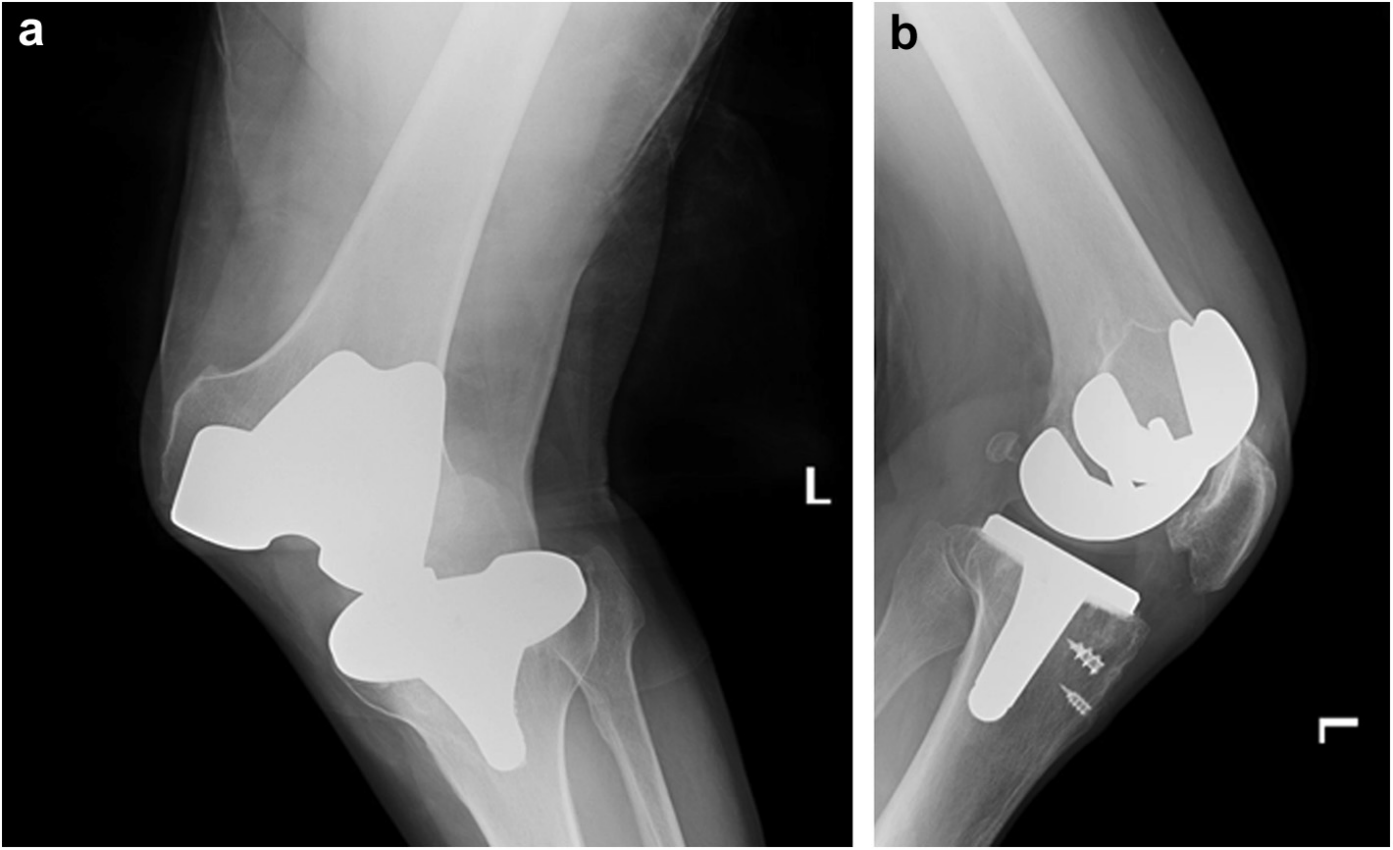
Anteroposterior (a) and lateral (b) radiographs demonstrating a left lateral TKA dislocation. Also noted are Arthrex Corkscrew FT anchors implanted at the time of primary TKA surgery.
